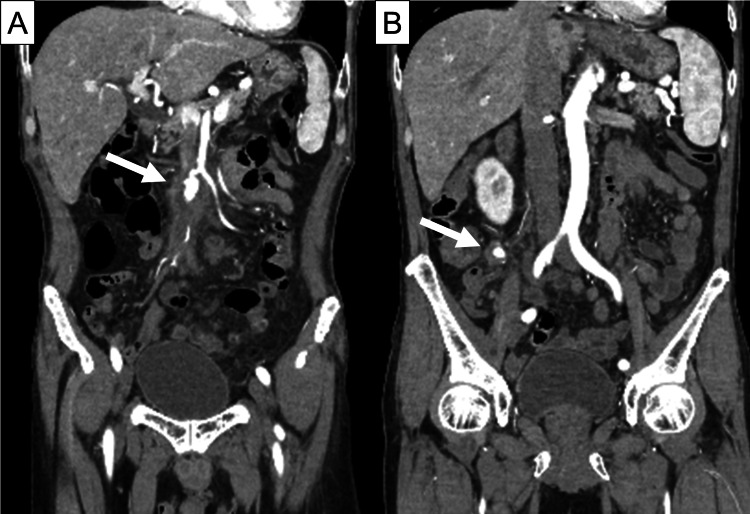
Abdominal contras-enhanced computed tomography (CT) on day 9. (A) Enlargement of the ileal artery aneurysm with surrounding low-density area (arrow), suggesting progression of the infection and necessitating urgent surgical intervention. (B) Mild enlargement of the ileocolic artery aneurysm (arrow) similarly indicated progression of the infection.
